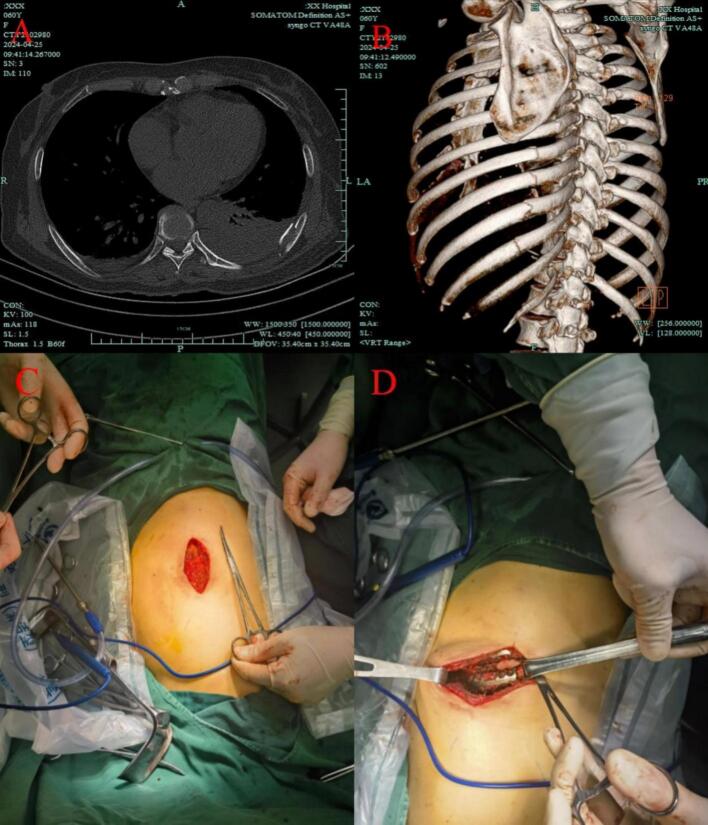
Surgical procedure. A: chest computed tomography; B: three - dimensional rib reconstruction; C: incision; D: titanium rib fixation plate.
